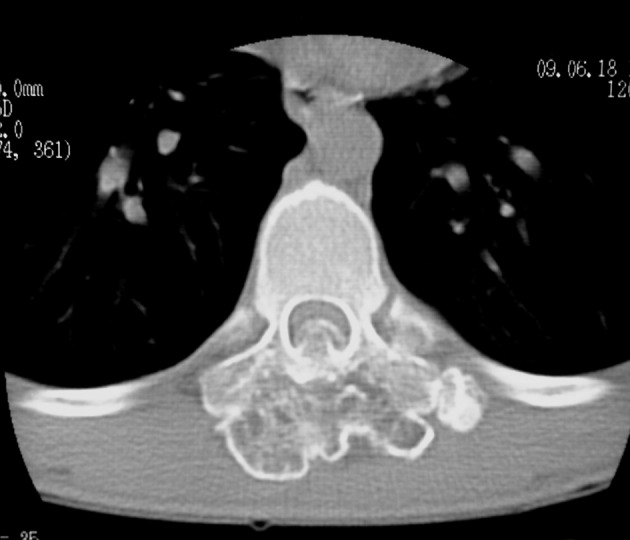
Bone window axial computed tomography scan at the level of T9 showing an abnormal bony mass arising from the posterior arch of T9 with extension to the spinal canal and compression of the spinal cord.
